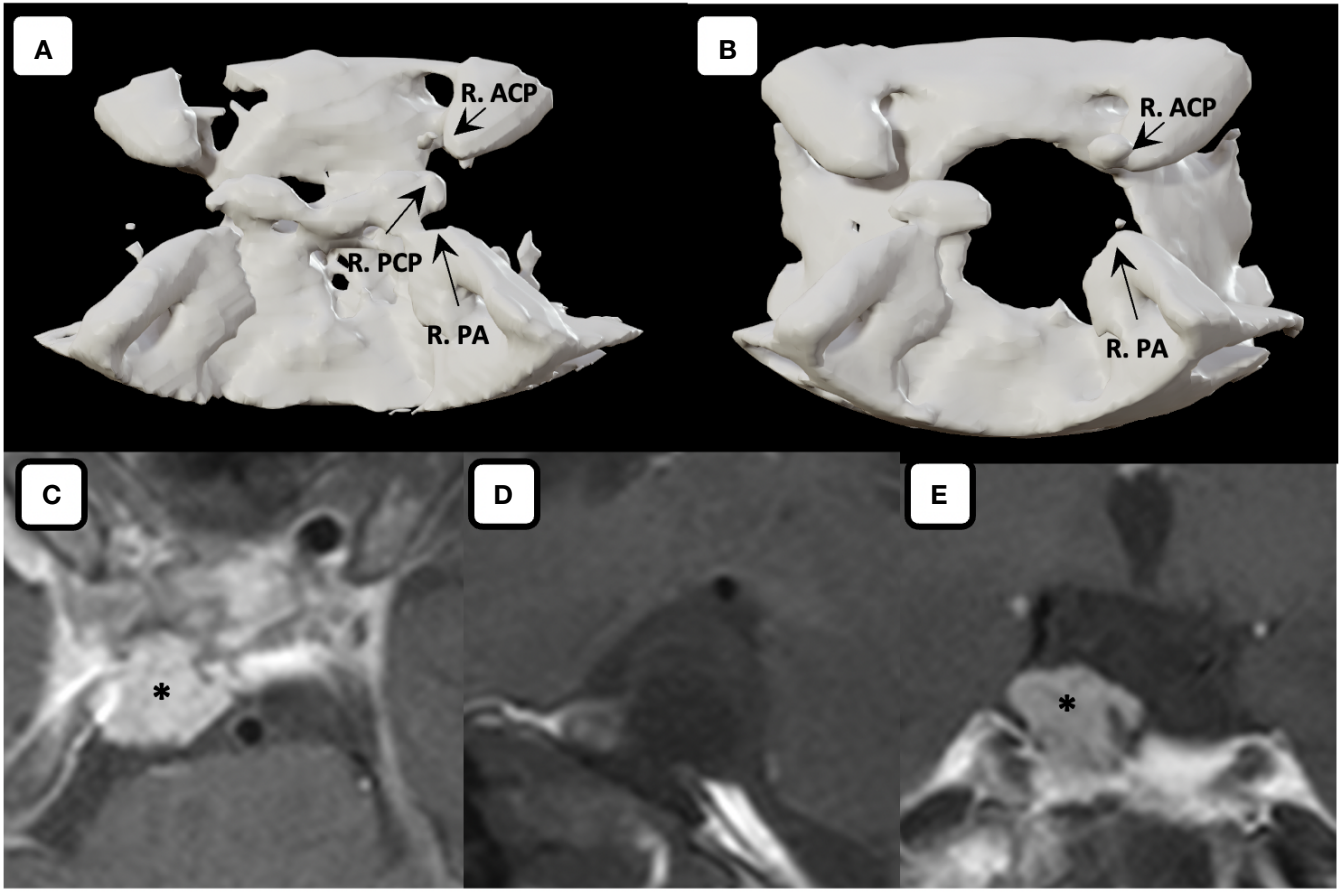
Three-dimensional computed tomography. Comparison between (A) preoperative and (B) postoperative three-dimensional computed tomography showed the skull base defect after performing right posterior clinoidectomy. Postoperative MR imaging of the tumor. (C) Axial, (D) sagittal (fat suppressed imaging), (E) coronal MR images showing gross total tumor removal are achieved with the fat graft covering the defects on the skull base without any sign of leakage. Asterisk denotes the fat graft on MR imaging. R. ACP, right anterior clinoid process; R. PA, right petrous apex; R. PCP, right posterior clinoid process.
